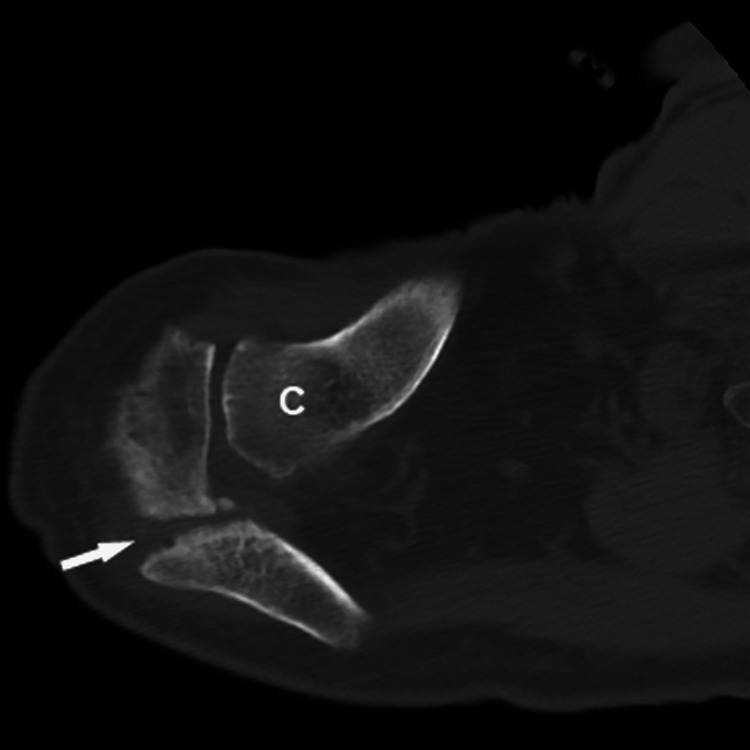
Axial CT scan of the right shoulder. Acromion fracture is indicated by the arrow; C, clavicle
